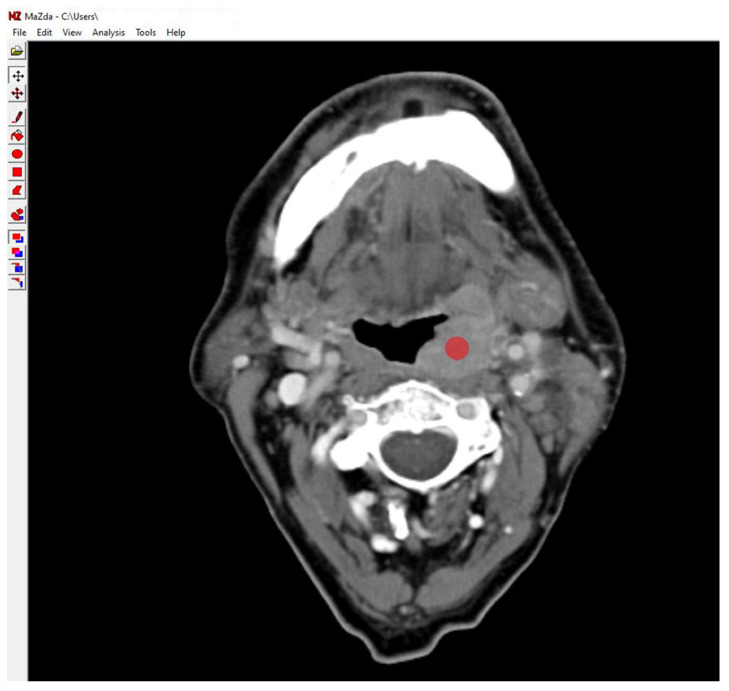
Example of contrast-enhanced axial multislice CT image displayed in the MaZda software interface, showing manual placement of a 5 mm circular region of interest (ROI) within a homogeneous area of the tumor.
